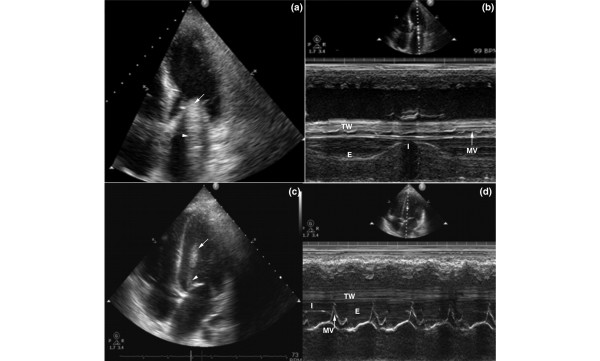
Echocardiography depicting the artifact (arrows) E, expiration; I, inspiration; MV, mitral valve; TW, thoracic wall. (a,c) Apical four-chamber views and (b,d) M-mode of the artifact in the left ventricle. The immobile part (arrow) and the mobile one (arrowhead) may be observed. The respiratory variation that resembles the lung 'sinusoid sign' is either fully (panel b) or partially (panel d) visible.
